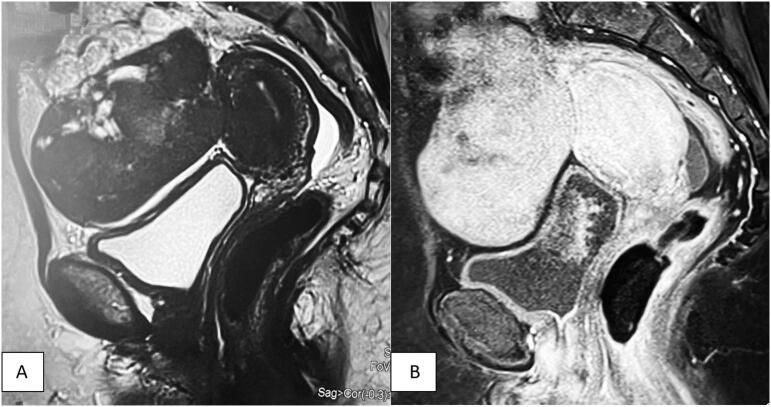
Magnetic resonance imaging of abdomen and pelvis. A: Coronal section of MRI showing the mass well defined heterogeneous in appearance with cystic areas. B: an MRI showing the enhancement after injection of the contrast.
